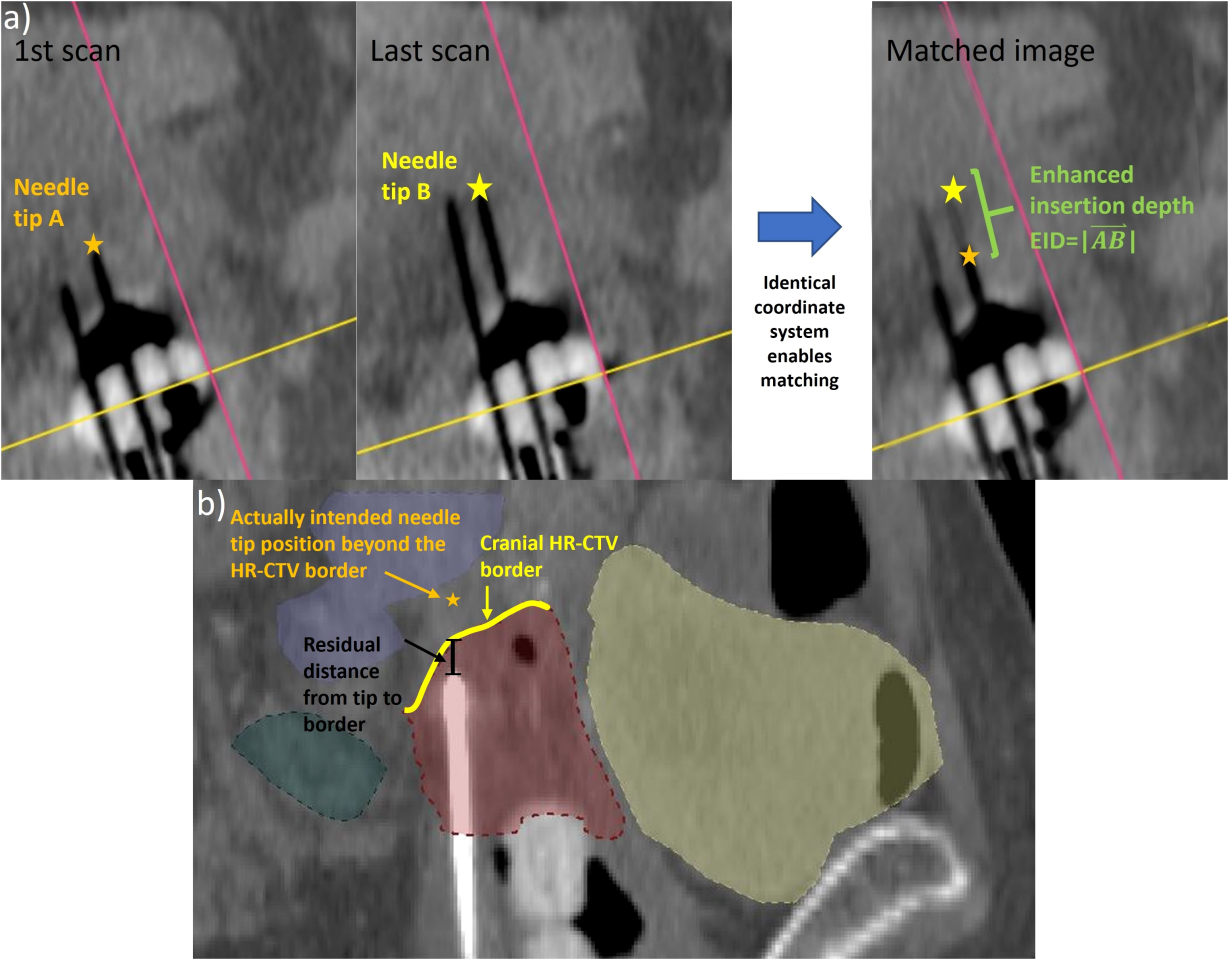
To determine the enhanced insertion depth (EID) as a measure for the applicator shift performed based on the intraoperative cone-beam computed tomography (CBCT) scans (a), the tip of each varied applicator was identified on the first and last intraoperative CBCT scans. Since the images were acquired in the same coordinate system, the EID could be determined directly from a corresponding match. Furthermore, the residual distance of each applicator that was not implanted to the cranial high-risk clinical target volume (HR-CTV) border (b) to this border along the implantation direction was calculated as a measure of implant inaccuracies.
